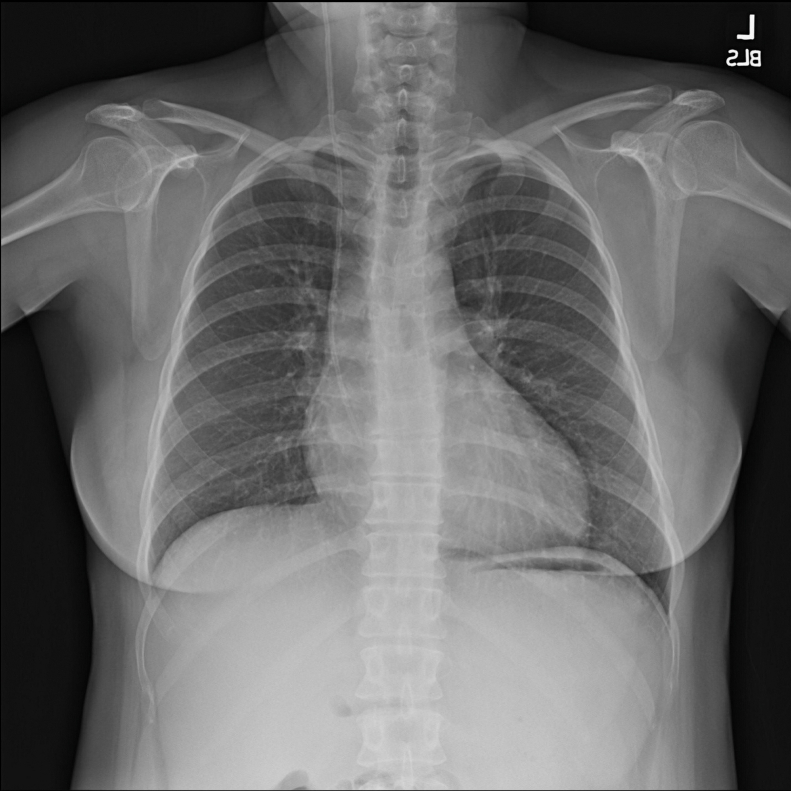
Chest radiograph demonstrating ventriculoatrial shunt tubing terminating in the right atrium.
